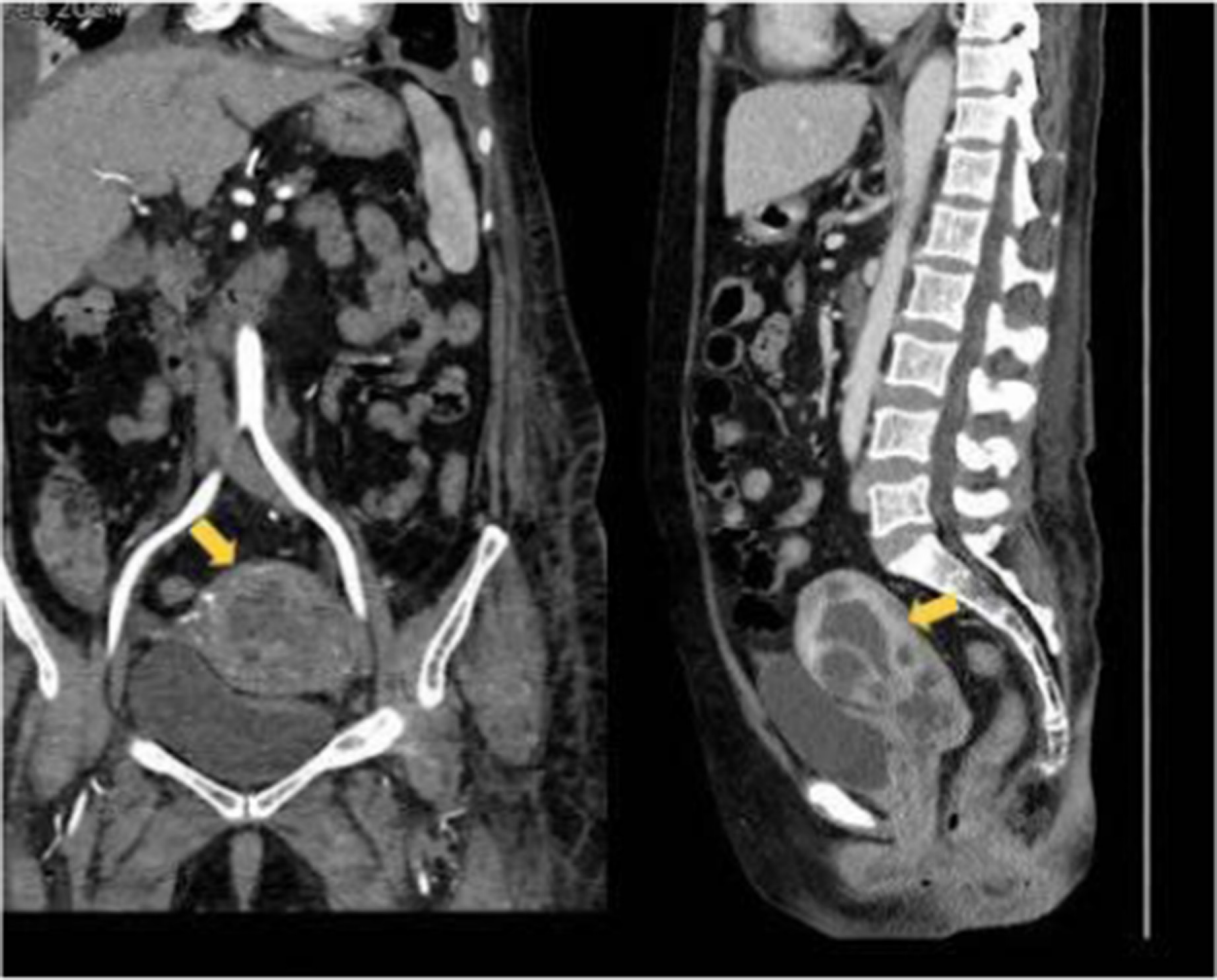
Coronal and Sagittal CT scans of the lower abdominal region. The yellow arrows indicates the 32 × 45 × 27 mm intramural mass on the posterior cervix wall, with infiltrative extensions into the lateral and anterior walls.
